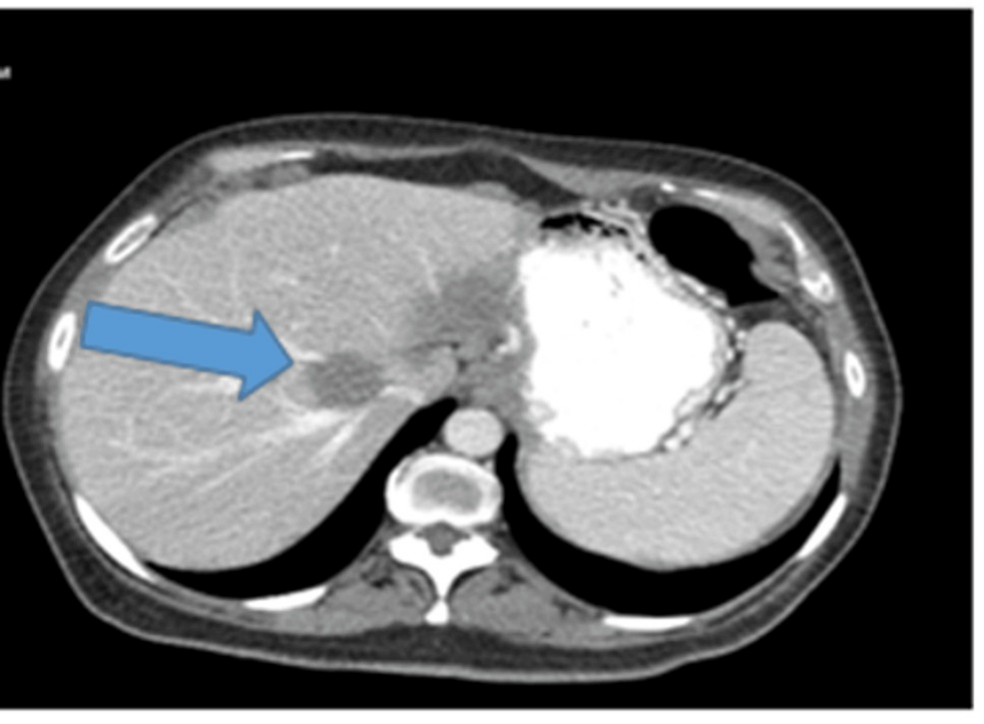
Chest CT abdominal with contrast showed new liver metastasis. CT: computed tomography
